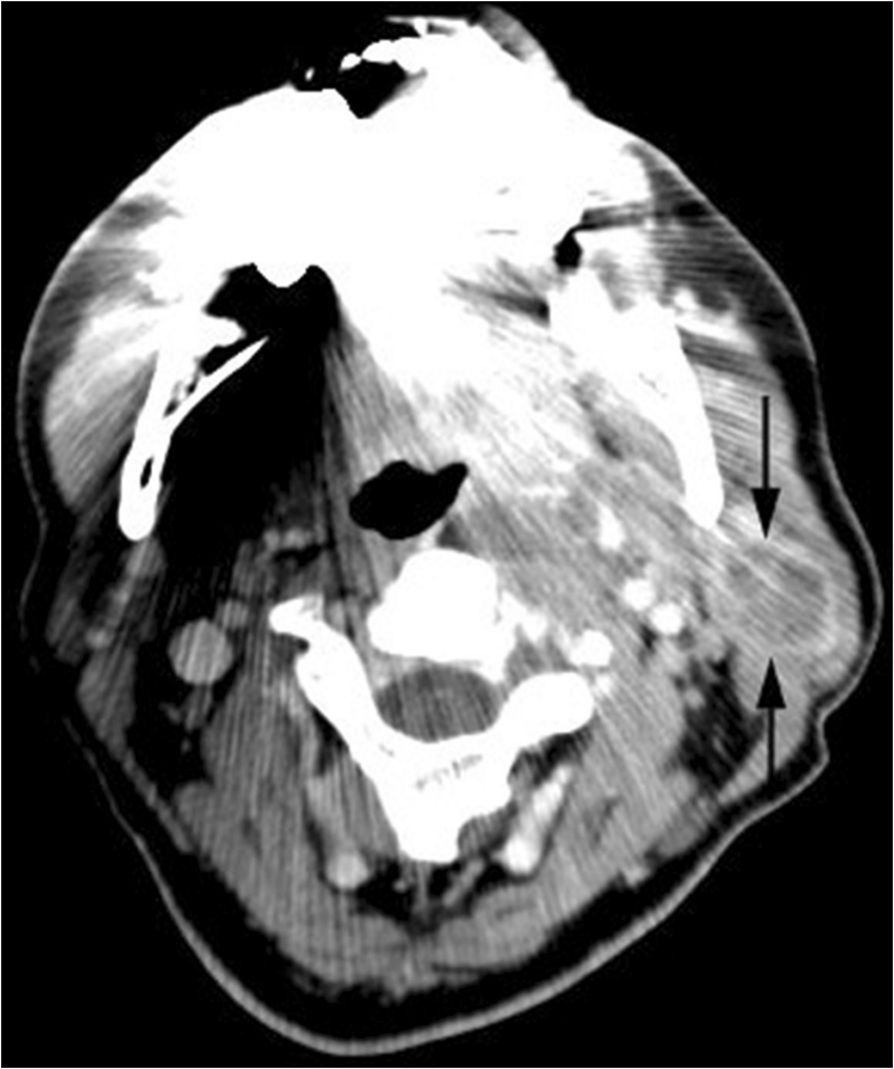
Computerized tomography (CT) at the level of the submandibular area demonstrating the mass of the left parotid gland extending into the carotid space.
